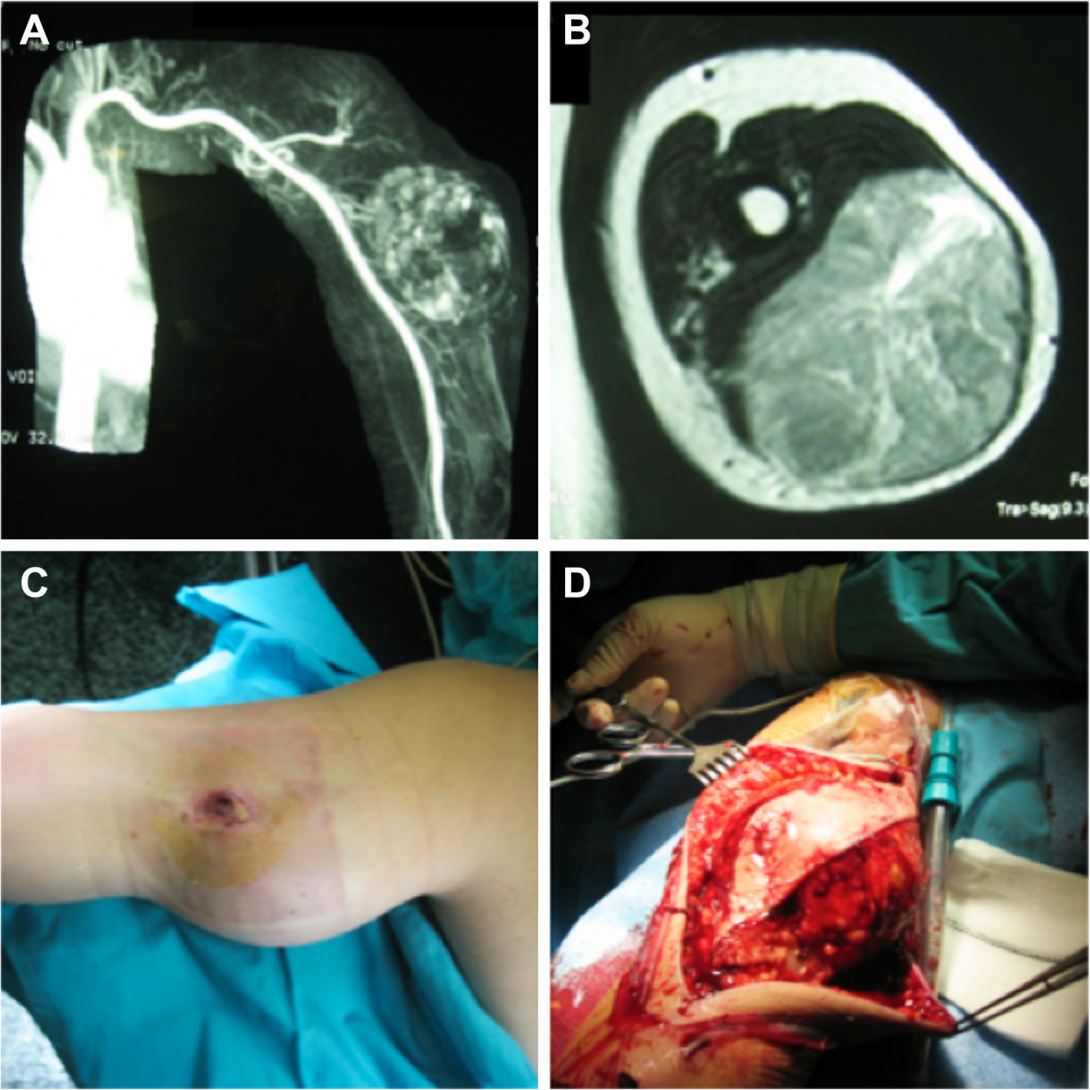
A 53-year-old female with grade III round cell pleomorphic liposarcoma (highly malignant) located in the triceps muscle of the left upper arm. a Resonance angiography showing an increase in tumour vascularization; b preoperative MRI, January 2007; c clinical appearance of the palpable mass; and d intraoperative appearance. The tumour measured 15 × 9 × 8 cm. This patient developed lung metastases and died 9 months after the diagnosis
